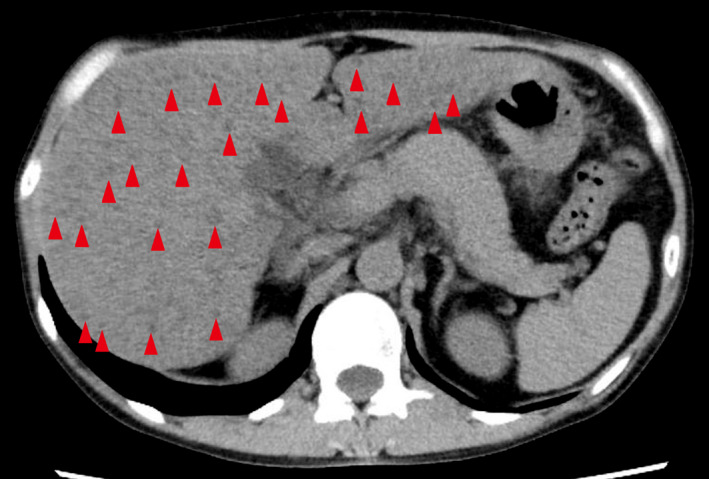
Abdominal computed tomography showing multiple hypodense liver masses
